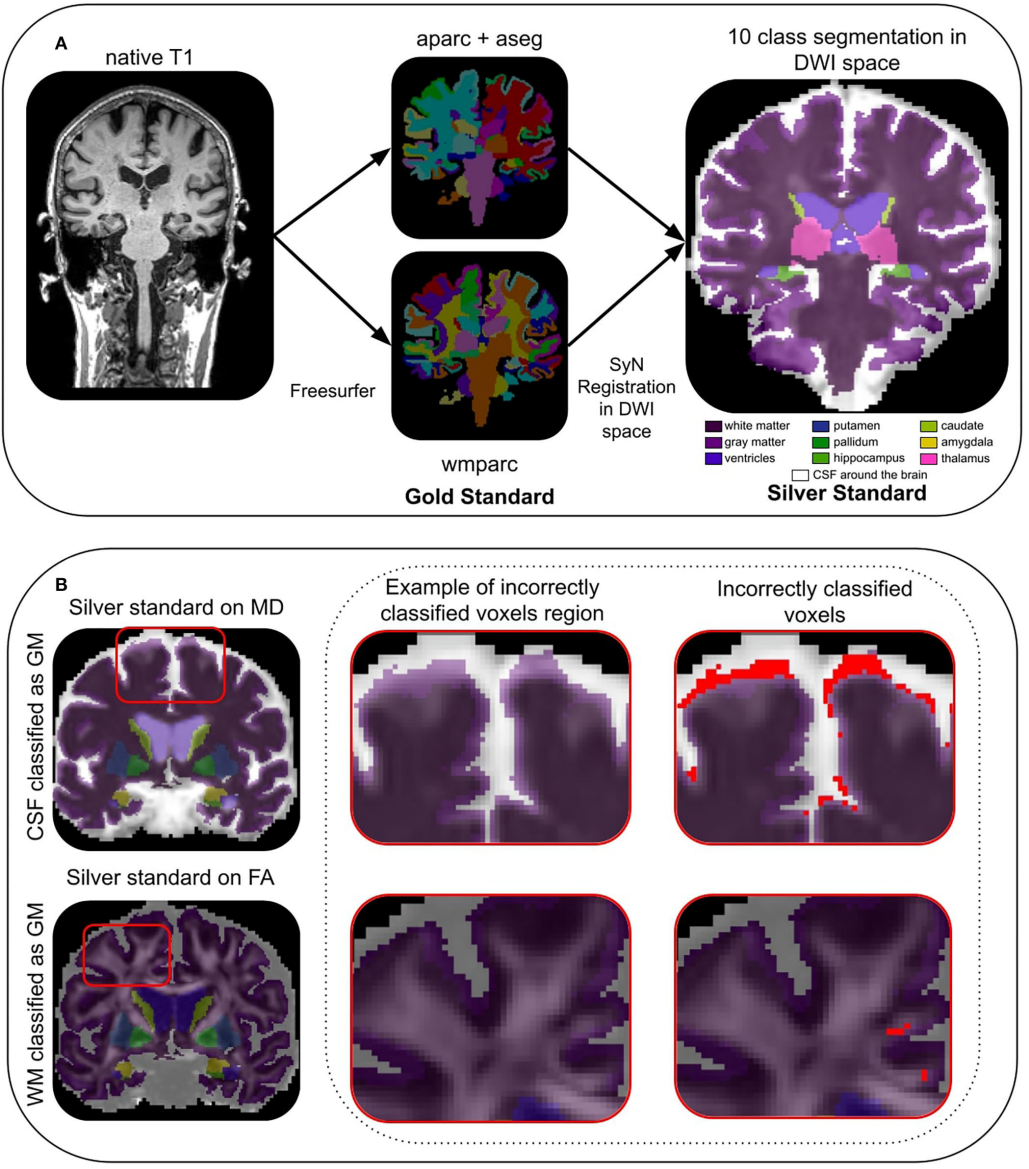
In (A), the process of generating the silver standard in DWI space from Freesurfer computed in the native T1 space. In (B), an example of CSF (row 1) and WM (row 2) incorrectly classified as GM (in red), in the last column.
